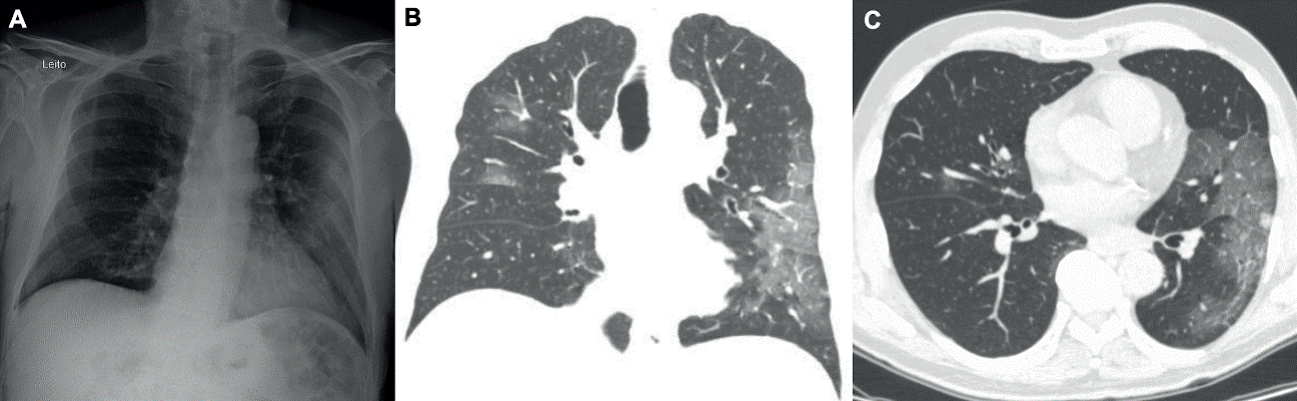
(A) A posteroanterior chest radiograph demonstrating ill-defined lung opacities, notably in the left lung. Chest CT images (lung window) in the coronal (B) and axial (C) planes show predominantly peripheral ground glass-opacities involving all pulmonary lobes, which are more exuberant in the left lung, where small foci of consolidation are also visible.
